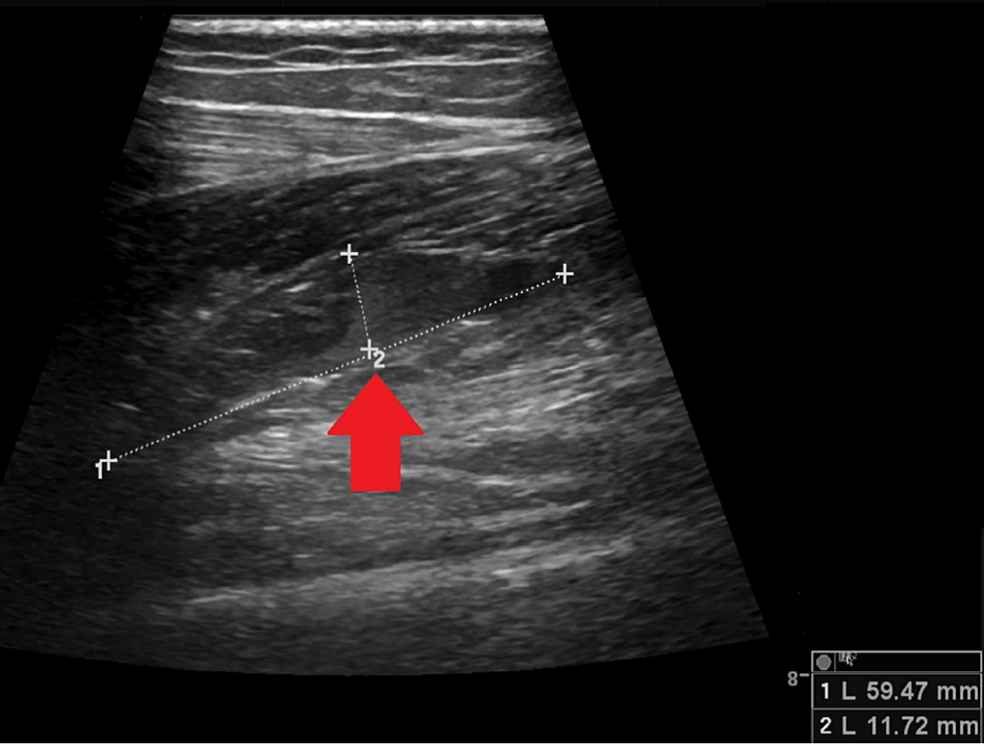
Lower limb Doppler imaging Acute venous thromboembolism (arrow) in the left popliteal vein
